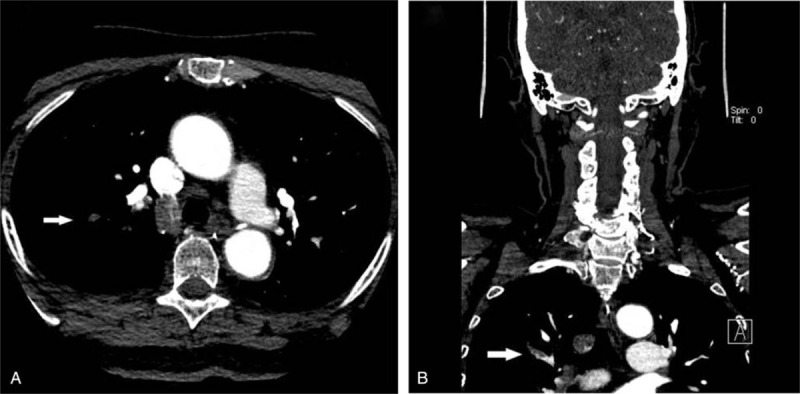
Axial (A) and coronal (B) CTA images demonstrating a pulmonary embolism within right upper lobe pulmonary artery (white arrow). This incidental finding was not initially reported on neck CTA.
